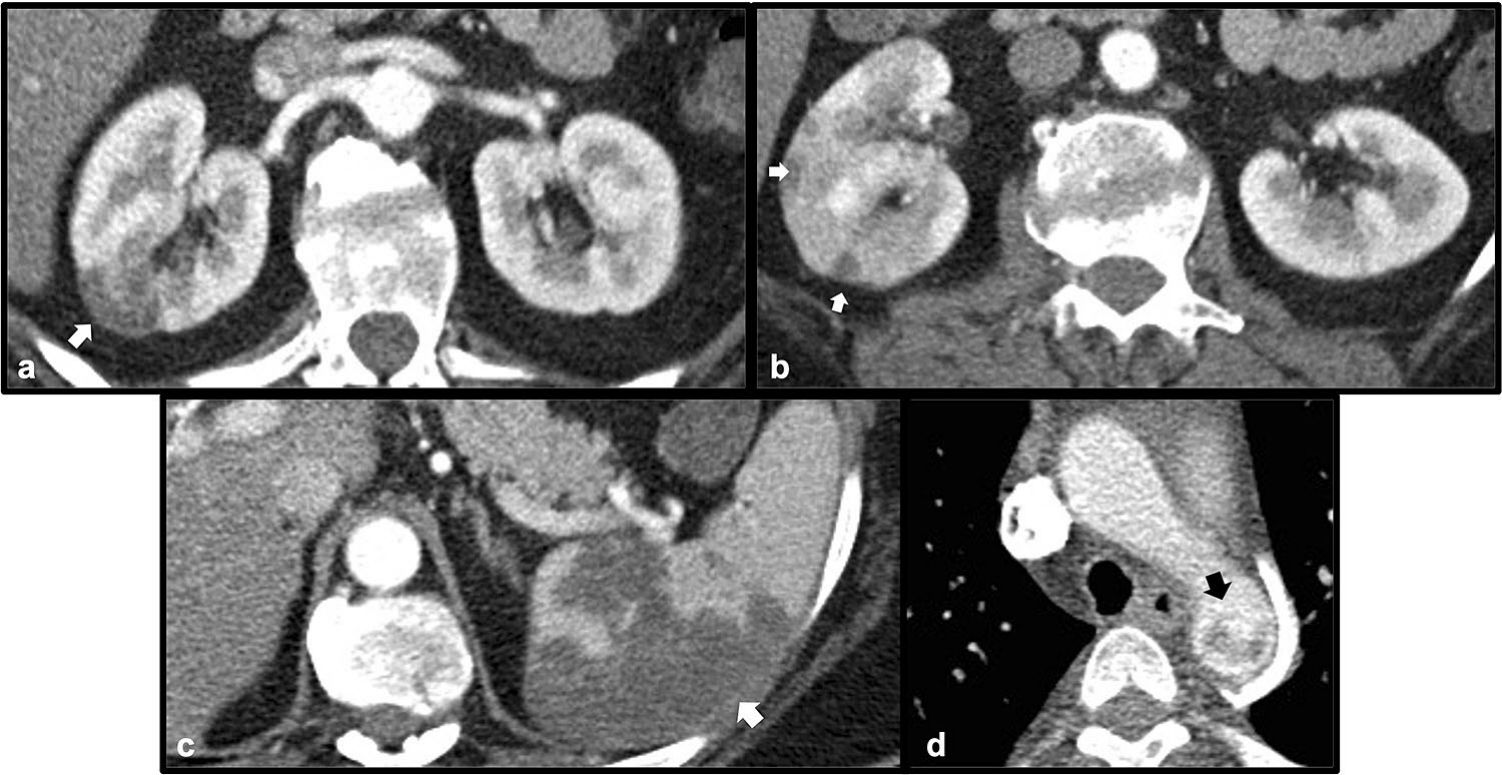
Axial post-contrast CT images (a-b) demonstrate multiple peripheral areas of low attenuation in the right kidney consistent with infarcts (white arrows). Axial post-contrast CT (c) demonstrates an additional infarct in the spleen (white arrow). A filling defect in the proximal descending thoracic aorta (black arrow) confirms mural aortic thrombus as the embolic source in this patient with atrial fibrillation
